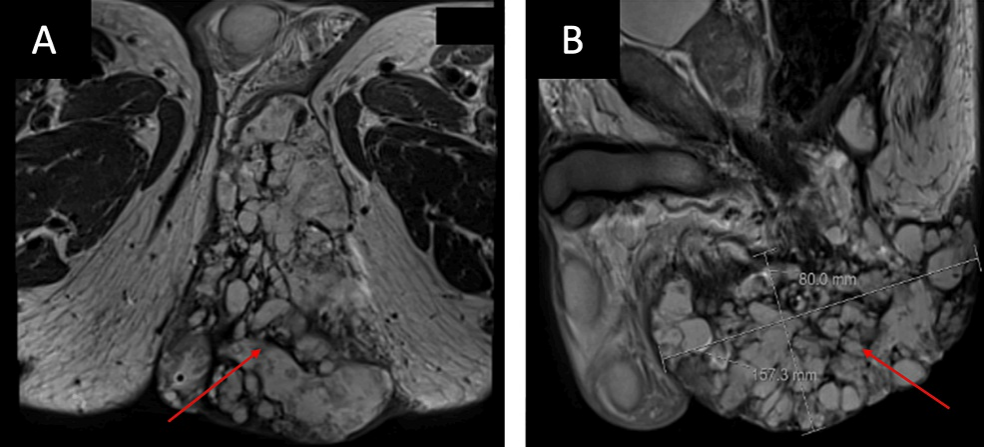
An axial (A) and sagittal (B) view of the pelvic magnetic resonance imaging showing a large T2 hyperintense, locally invasive, heterogeneous tumor (red arrow) infiltrating the posterior wall of the anal canal and lateral walls of the lower rectum.
